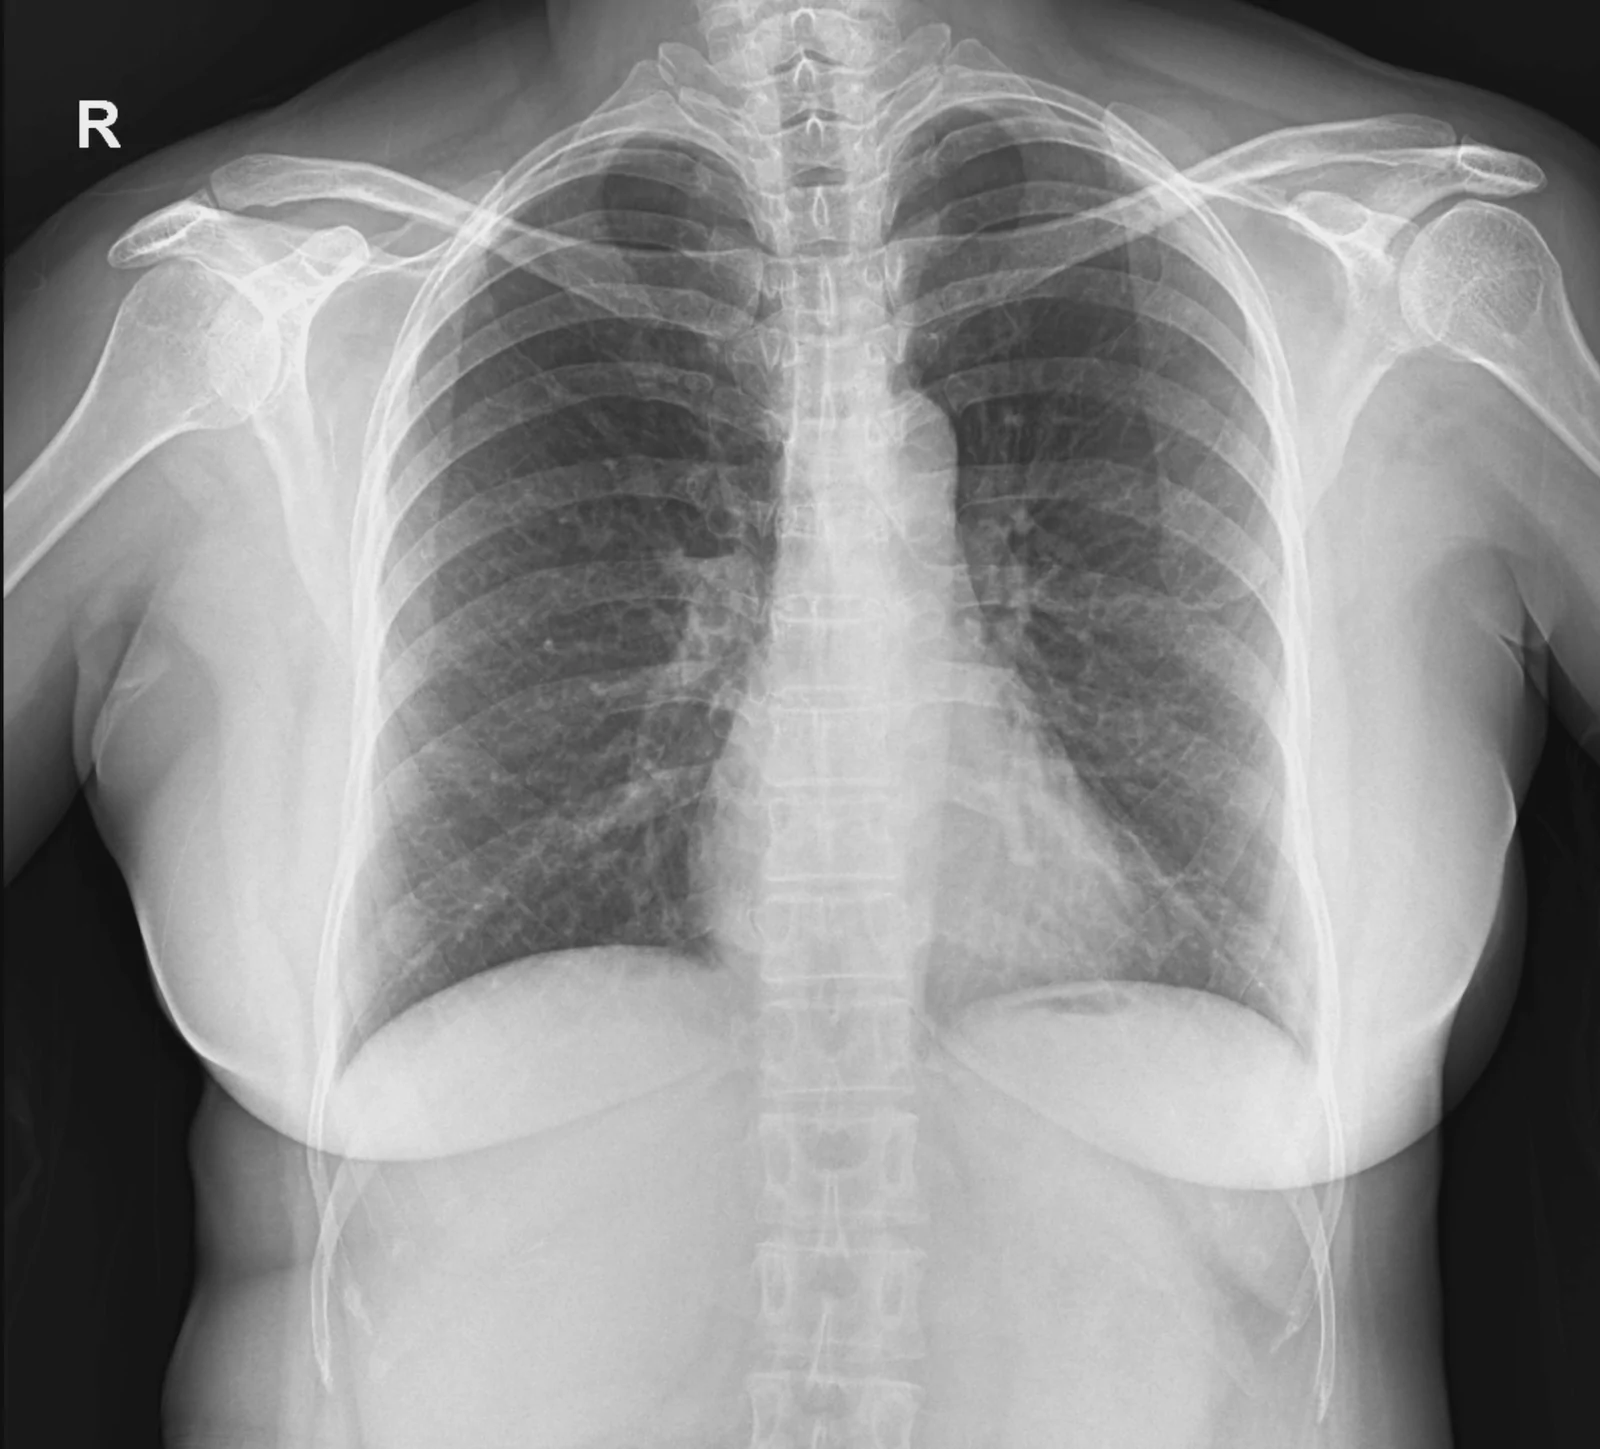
Posteroanterior chest X-ray showing no obvious abnormality. The patient’s chest X-ray shows no evidence of focal consolidation, pleural thickening, or pleural effusion. No radiographic features suggestive of pulmonary tuberculosis are seen.
